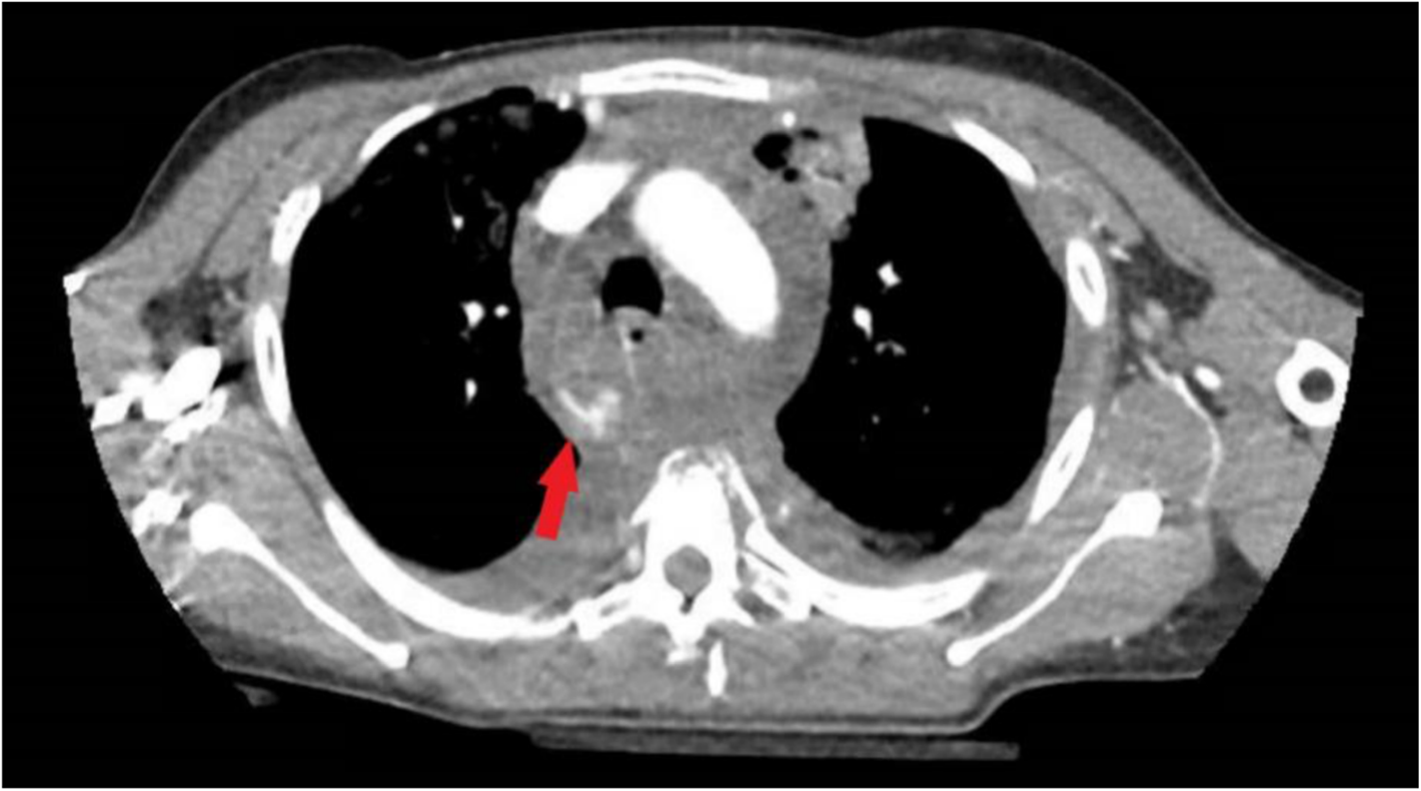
Axial CT slice through the azygous venous arch showing active extravasation of contrast (arrow) from the inferior aspect of the arch
